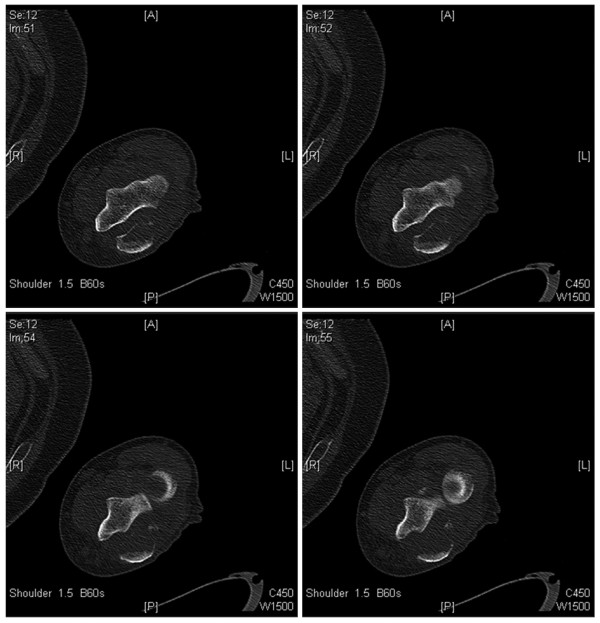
Preoperative radiograph of the left proximal ulna and pathologic fracture of the olecranon.
